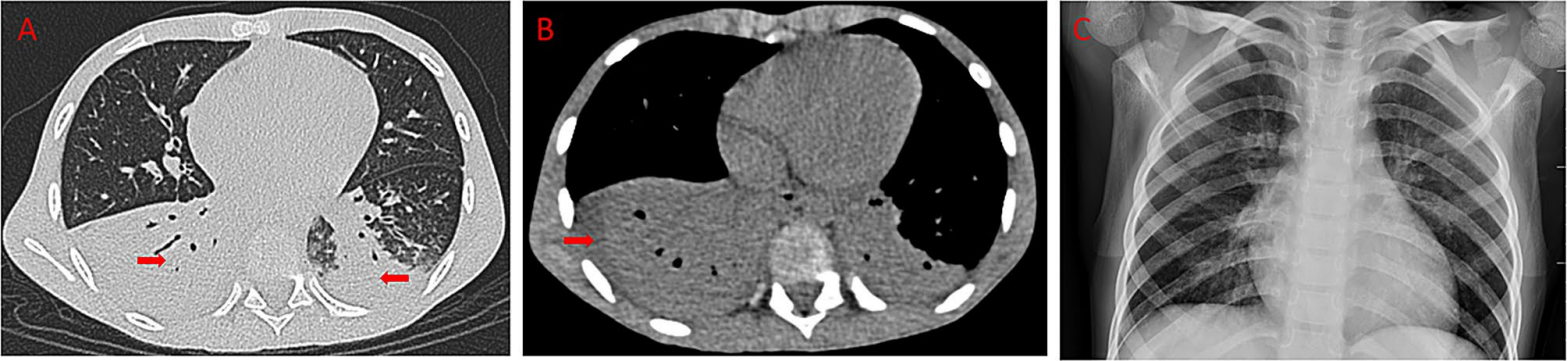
Chest imaging before and after treatment. A: Pre-treatment chest CT (lung window) shows partial consolidation in the lower lobes of both lungs (as indicated by red arrows). B: Pre-treatment chest CT (mediastinal window) reveals moderate bilateral pleural effusion and partial compressive atelectasis of the adjacent lower lobes (as indicated by red arrows). C: CXR at 1-month follow-up after discharge demonstrates resolution of pulmonary inflammation with no new lesions
